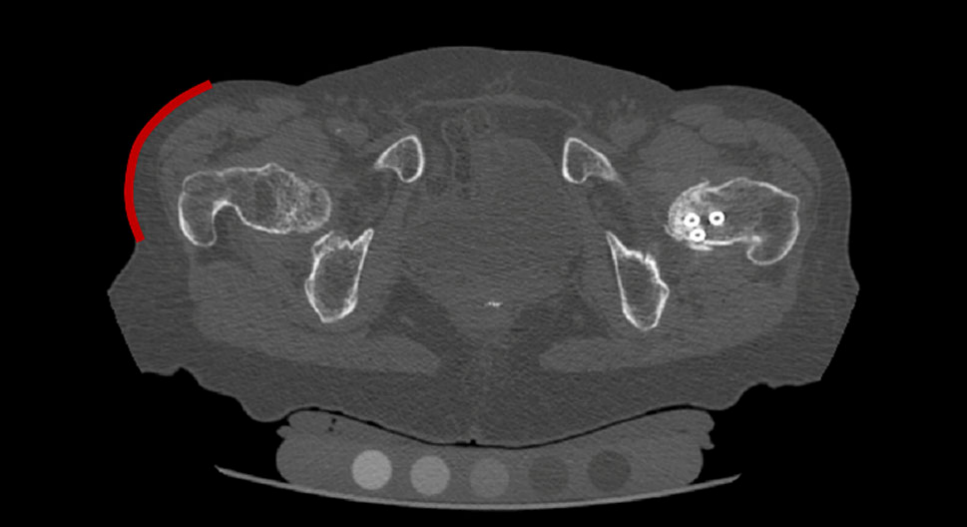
One CT slice with the pelvic surface highlighted in red. The surface was segmented in order to locate the end of the soft tissues surrounding the distal femur and measure the STT along the different orientations. The full soft-tissue profile is built by segmenting each CT slice in the image-set.
